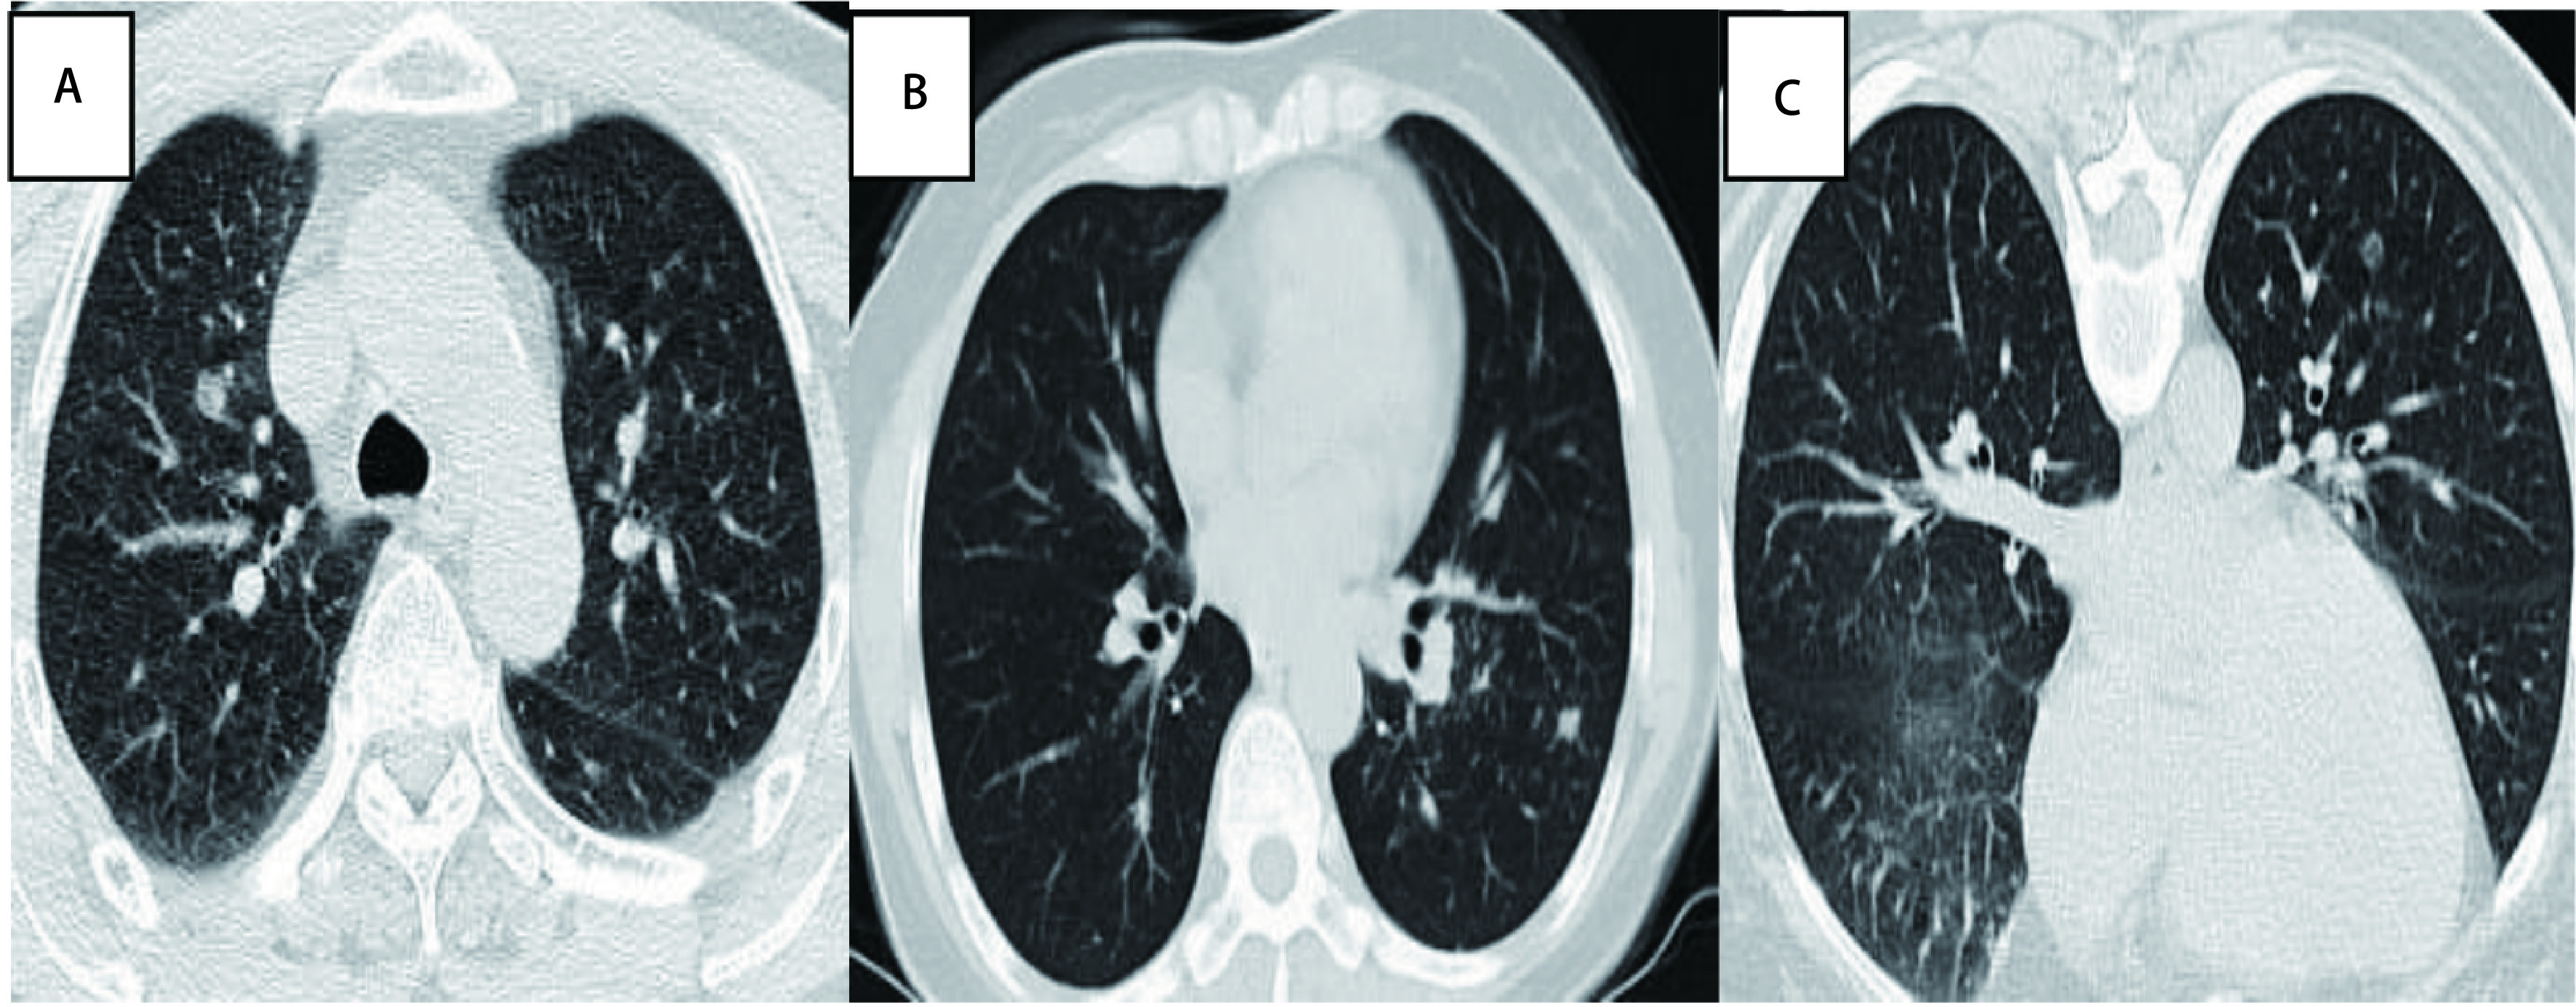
患者CT征象。A：右肺上叶实性小结节；B：左肺下叶亚厘米结节；C：左肺下叶亚厘米磨玻璃结节 CT signs of patients. A: right upper lobe solid nodules; B: left lung nodules; C: left lower lobe sub-centimeter ground-glass nodules. CT: computed tomography.
